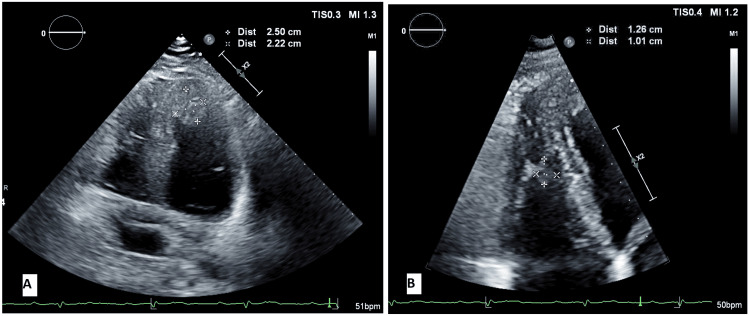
Case one echocardiogram showing biventricular thrombi (A) The left ventricular thrombus measuring 2.50 x 2.22 cm and (B) The right ventricular thrombus measuring 1.26 x 1.01 cm.
